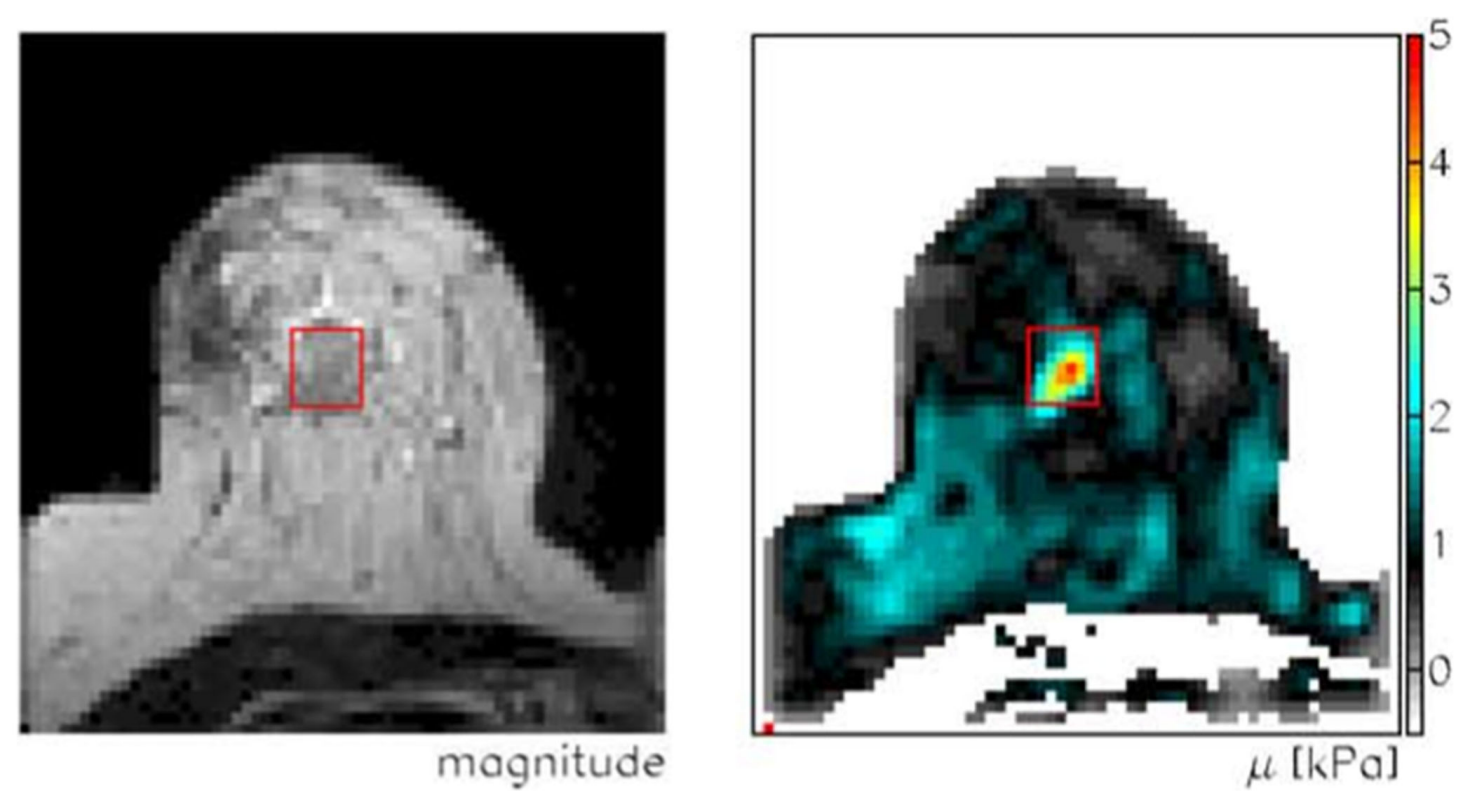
Magnetic resonance imaging (left) and elastography (right) of a breast tumour. The tumour (red rectangle) is stiffer than its surroundings. Reprinted from [8], Copyright 2005, with permission from Elsevier.
